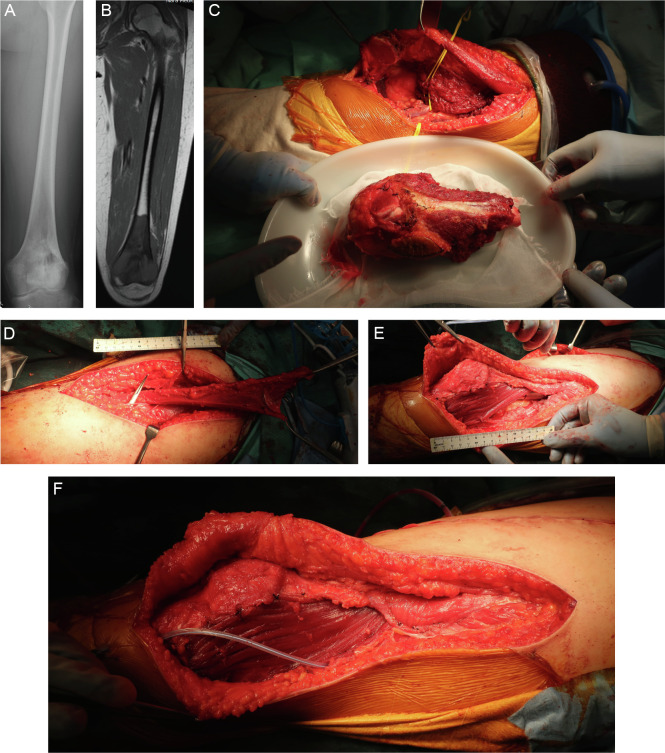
Case 1: 15-year-old female patient. (A, B) An incisional biopsy was performed from the lateral side of the distal femur, and a conventional osteosarcoma in the distal part of the left femur was diagnosed. No distant metastasis was found at the time of the initial diagnosis. Preoperative chemotherapy (methotrexate + doxorubicin + cisplatin regimen) was administered. A preoperative computed tomography scan of the chest revealed multiple lung metastases. (C–F) Wide resection including the skin around the biopsy site, reconstruction with a megaprosthesis, and sartorius muscle flap coverage were performed. After the surgery, the lung metastases were inoperable, and ifosfamide + etoposide, pazopanib, and gemcitabine + docetaxel were administered. However, the lung metastasis could not be controlled, and the patient died 1 year 7 months after the diagnosis.
